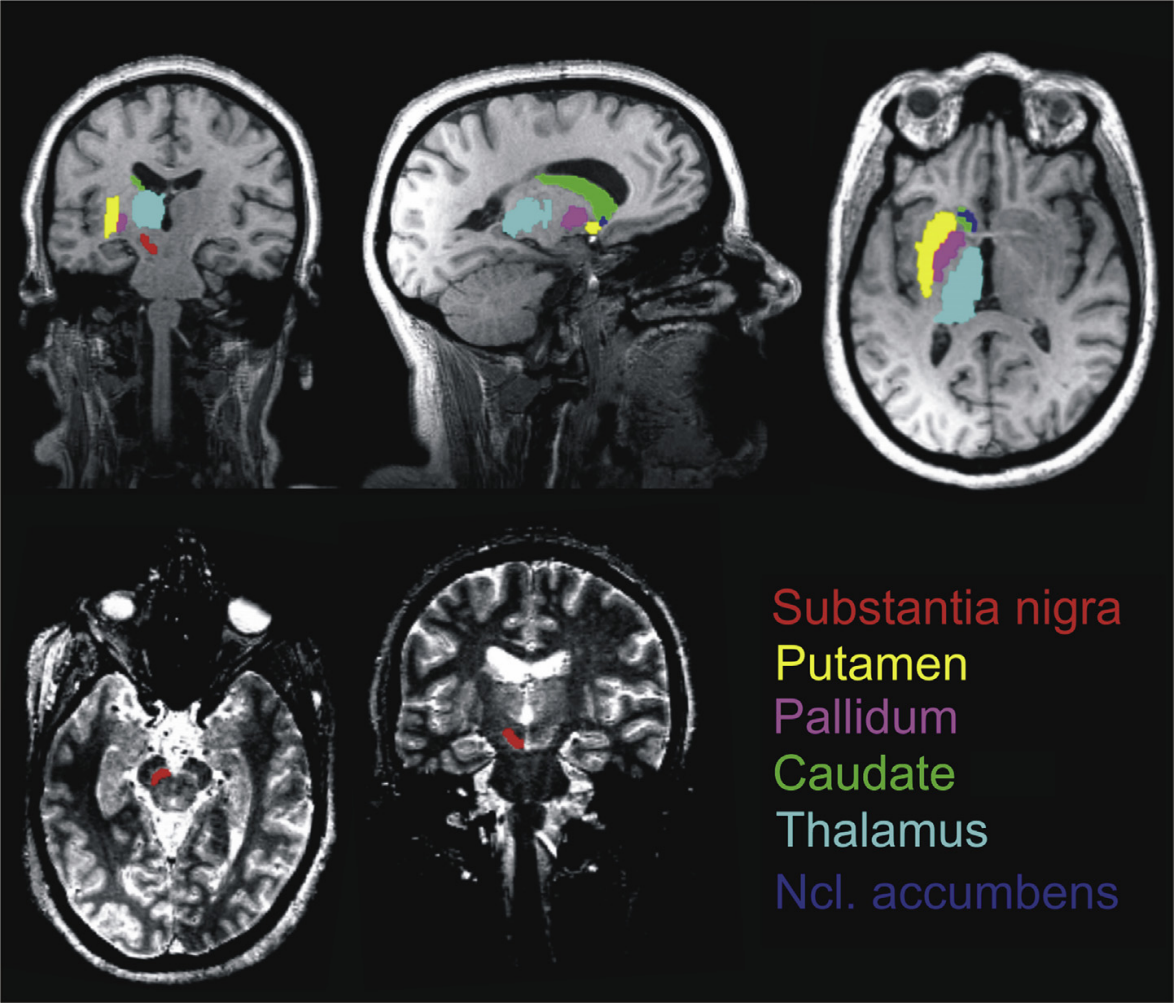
Regions of interest (ROI). The SN was defined manually for each subject. All other ROIs were segmented automatically (see Methods). Upper row shows left hemisphere ROIs overlaid on an individual T1-weighted image and lower row shows the subject's MT-image.
